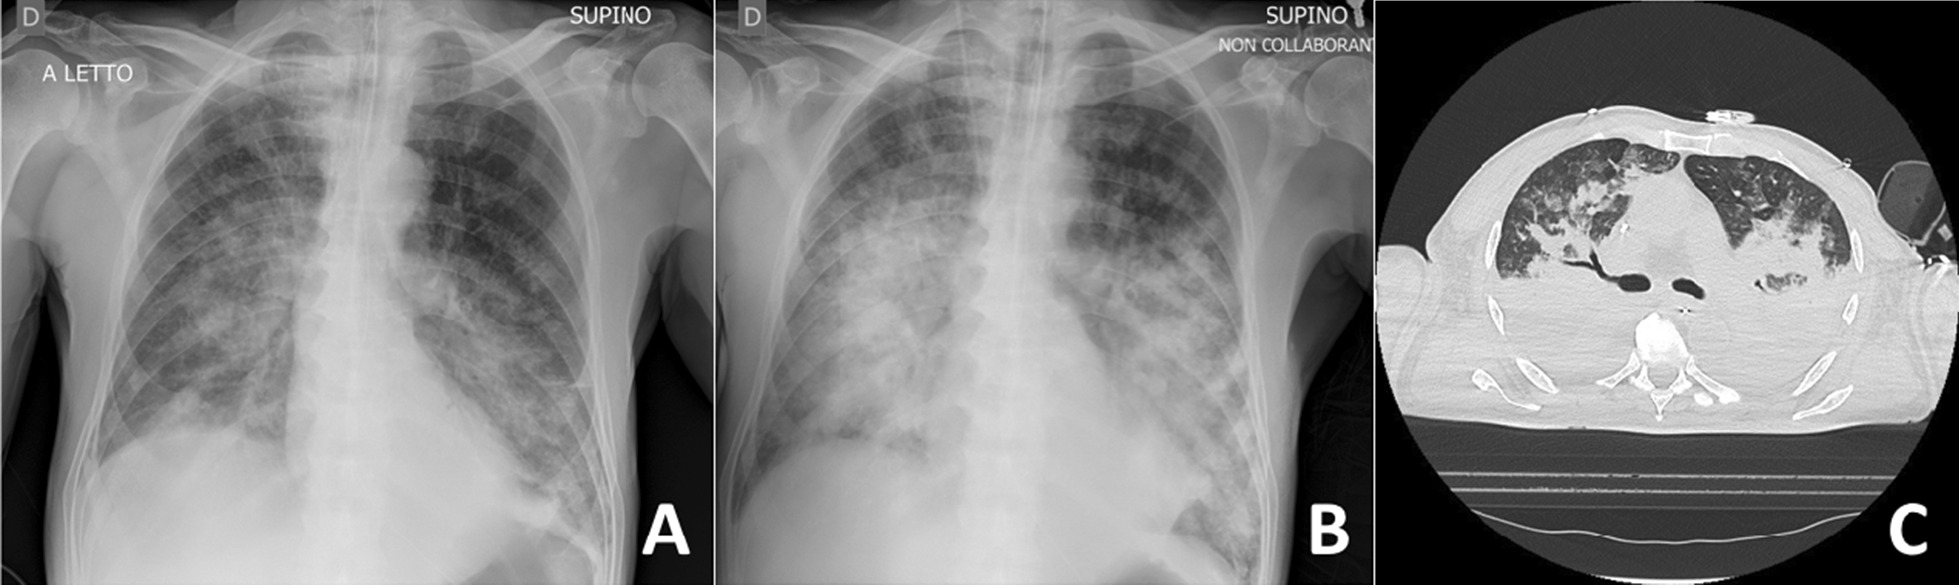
A Chest X-ray performed immediately after massive aspiration. B Chest X-ray 4 hours later in a level-2 hospital intensive care unit. C Lung computed tomography after initiation of veno-venous extracorporeal membrane oxygenation and clinical improvement in the level-1 hospital
